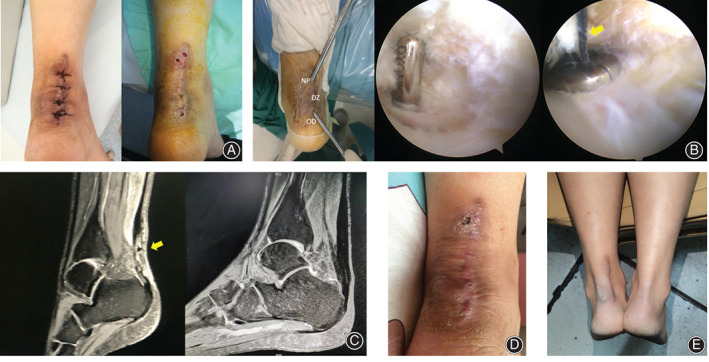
(A) The situation after Achilles tendon suture repair and the wound situation after one and a half months. (B) Intraoperative approach and arthroscopic findings. The yellow arrow indicates that the thread that caused delayed wound healing is being removed. (DZ, delayed wound healing zone; NP, new portal; OD, original defect.) (C) Preoperative and postoperative (6 months later) Achilles tendon MRI. The arrow indicates a high‐signal area, which, considering the patient's delayed wound healing and inflammation, may represent foreign body response caused by a suture. (D) The wound 1 week after surgery. (E) The current wound condition (4.5 years after surgery) which has completely healed.
